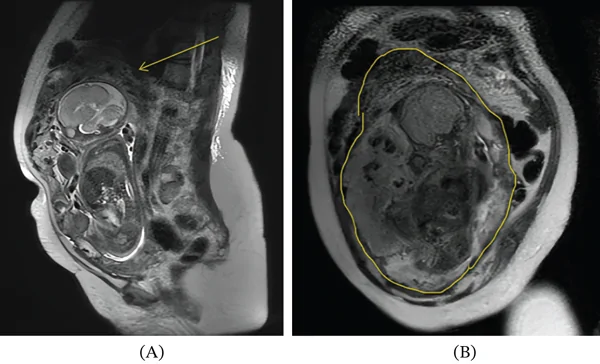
MRI demonstration of the low‐lying gestational configuration. (A) Sagittal T2‐weighted MR image showing a prominent fundal myometrial mantle with no well‐defined gestational sac in the expected fundal uterine cavity (arrow). (B) Coronal T2‐weighted MR image delineating the pear‐shaped uterine configuration and the low‐lying position of the gestational sac.
